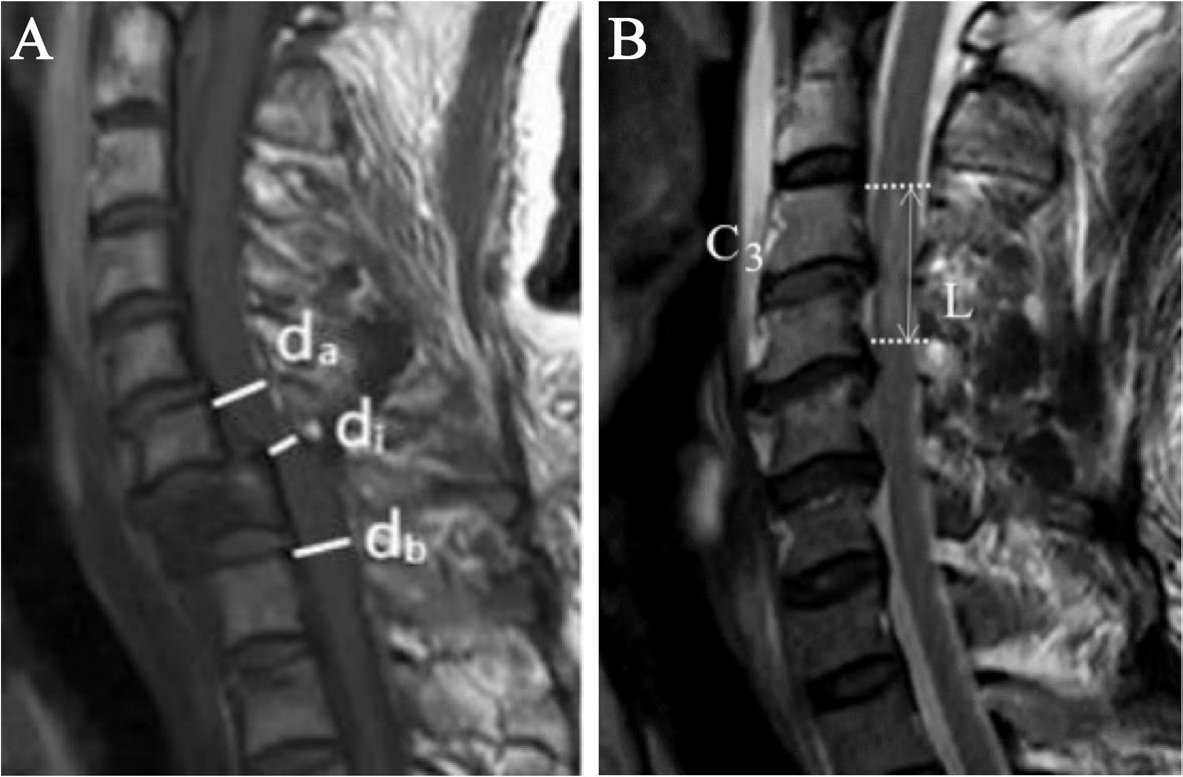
Measurement methods of MCC and IMLL. Abbreviations: MCC: maximum canal compromise; IMLL: intramedullary lesion length. Notes: (A) Select the median sagittal image of the magnetic resonance imaging, d_i_ represents the anteroposterior canal diameter of the maximum damage level, while d_a_ and d_b_ represent the diameter of anteroposterior canal diameter nearest to the normal level above and below the injury site, respectively. MCC (%) = 1-[ 2d_i_ / (d_a_ + d_b_)] × 100%. B Select the median sagittal image of the magnetic resonance imaging, and the rostrocaudal length of damage was measured in centimeters. The normal intramedullary signal was recorded as zero
